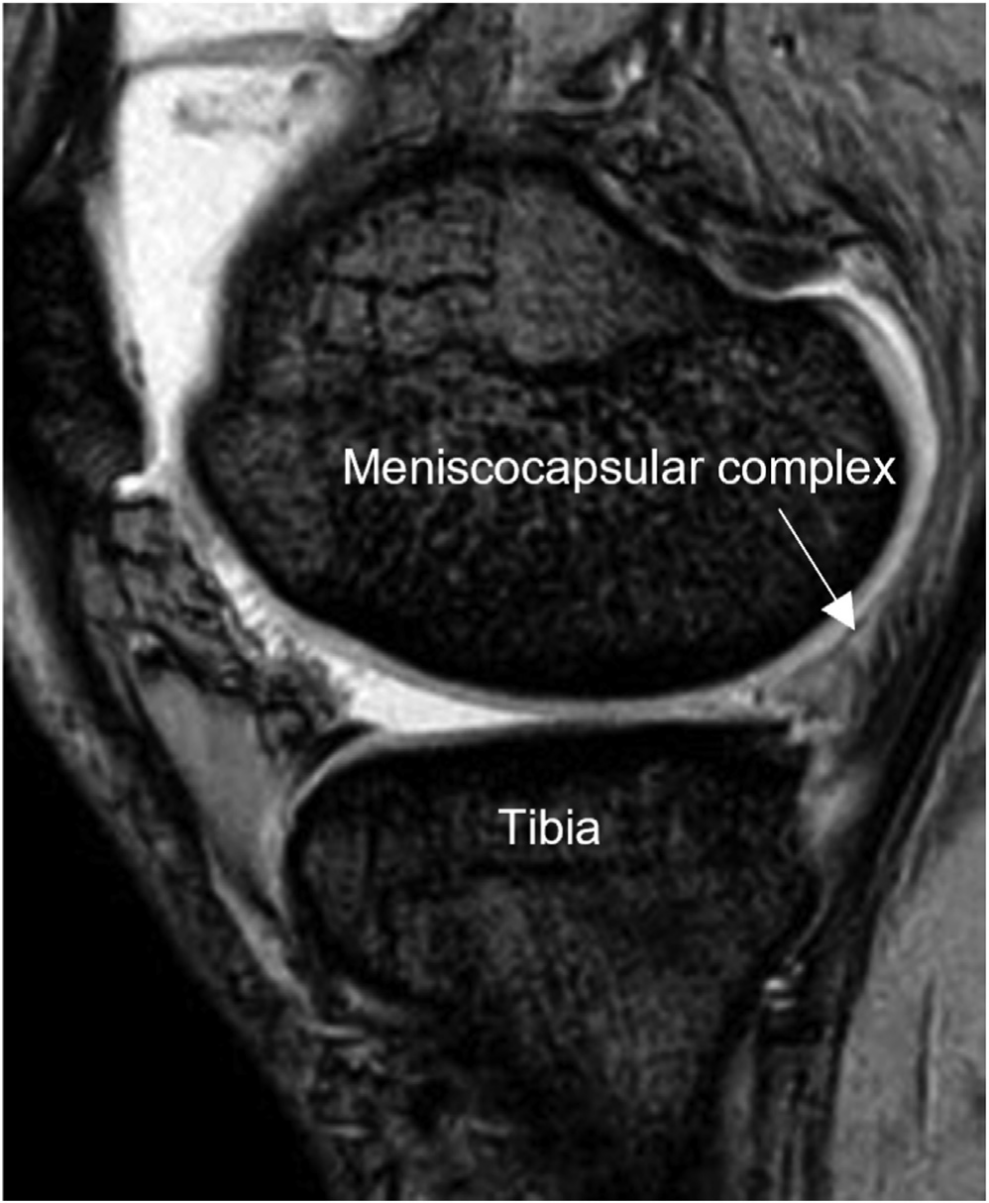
Sagittal magnetic resonance imaging of a left knee demonstrating posterior displacement of the meniscocapsular complex (white arrow) and anterior tibial subluxation.
